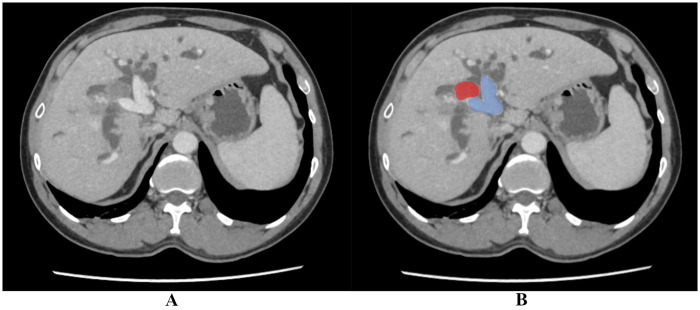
Preoperative MRI findings demonstrating the bismuth-corlette type 3A klatskin tumour. (A) Unannotated MRI showing a heterogeneous hilar mass at the confluence of the common hepatic duct with a patent main portal vein. (B) Annotated MRI with the tumour highlighted in red and the patent portal vein in blue.
